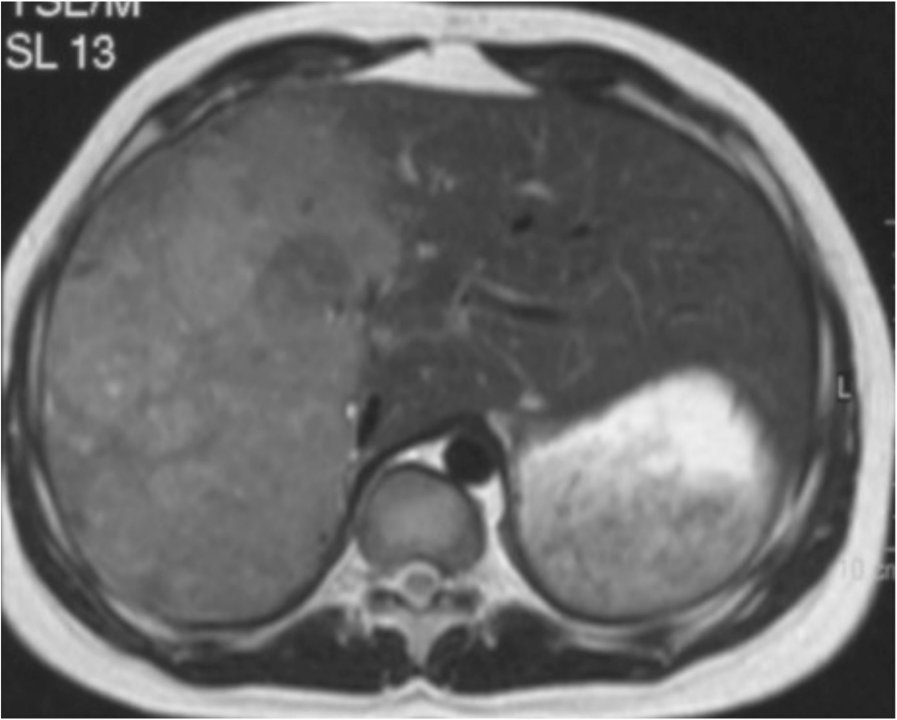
Image of magnetic resonance imaging scan showing a large mass in segments 4 to 8 of the liver
